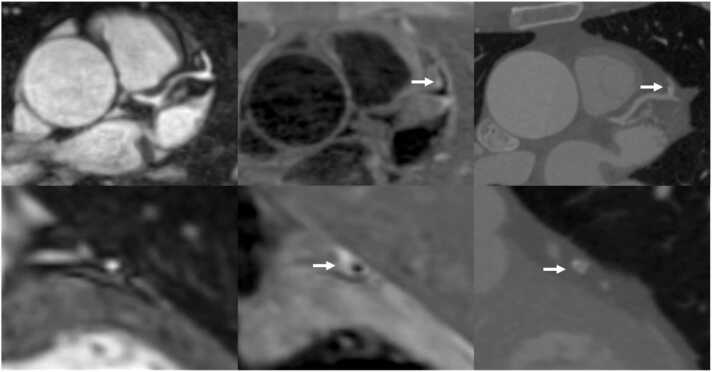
iT2prep-BOOST and CCTA assessment of a mid-LAD plaque. Top row: axial iT2prep-BOOST bright-blood image (left), black-blood image (center), and corresponding CCTA (right). Bottom row: cross-sectional views at the level of a plaque in the mid-LAD using the same modalities. White arrows indicate the location of the atherosclerotic plaque, which appears hyperintense on the black-blood image and corresponds to a partially calcified lesion on CCTA. *CCTA* coronary computed tomography angiography, *LAD* left anterior descending, *BOOST* bright‐blood and black‐blOOd phase SensiTive
